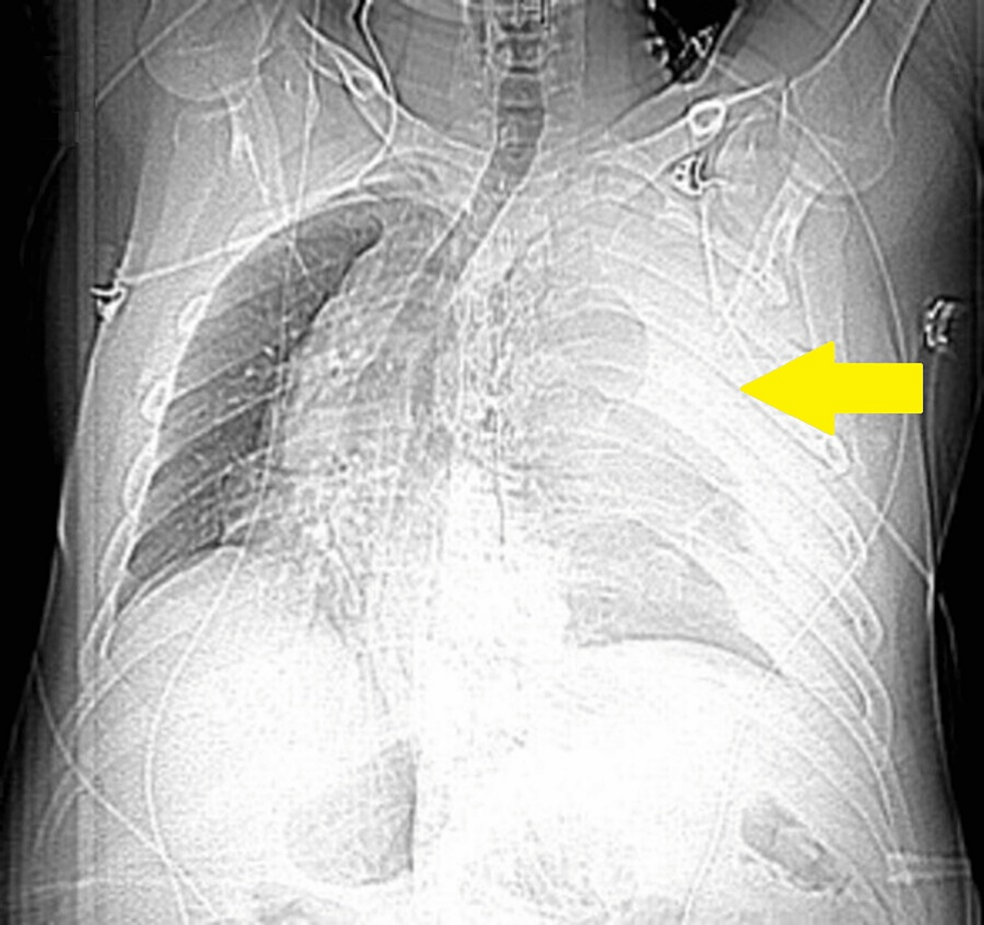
Chest X-ray demonstrating complete opacification of the left hemithorax with a right mediastinal shift.
